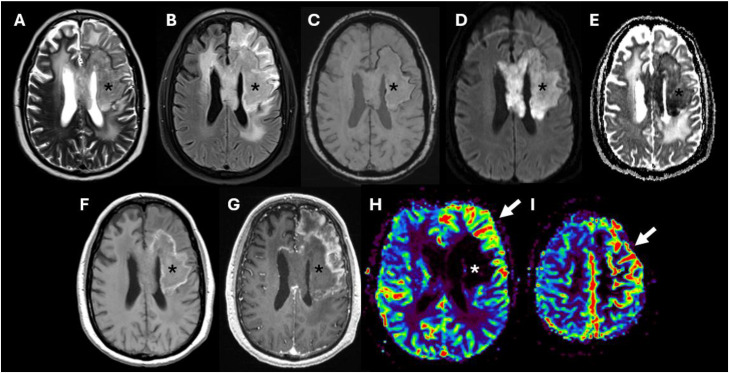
Two-month follow-up brain MRI demonstrating axial T2-weighted (A), FLAIR (B), susceptibility-weighted imaging (SWI) (C), diffusion-weighted imaging (DWI) (D), apparent diffusion coefficient (ADC) map (E), axial T1-weighted without contrast (F), axial post-contrast T1-weighted (G), and relative cerebral blood volume (rCBV) perfusion maps (H, I). At the time of imaging, anti-angiogenic therapy had been suspended for 2 months. Similar features of treatment-related necrosis (asterisks) with marked diffusion restriction on DWI with corresponding low ADC values. Additionally, new gyriform enhancement (G, white arrows) is identified in the left frontal lobe – superior, middle and pre central gyri -, associated with marked hyperperfusion on perfusion imaging (white arrows) and no diffusion restriction.
